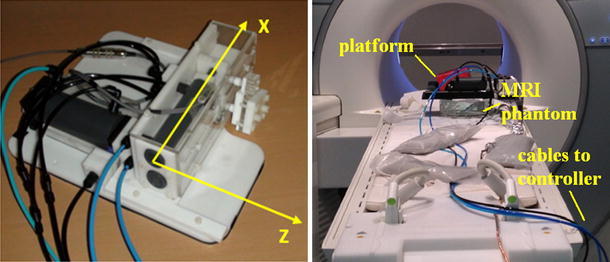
MRI-compatible pneumatic moving platform controlled remotely from control room
